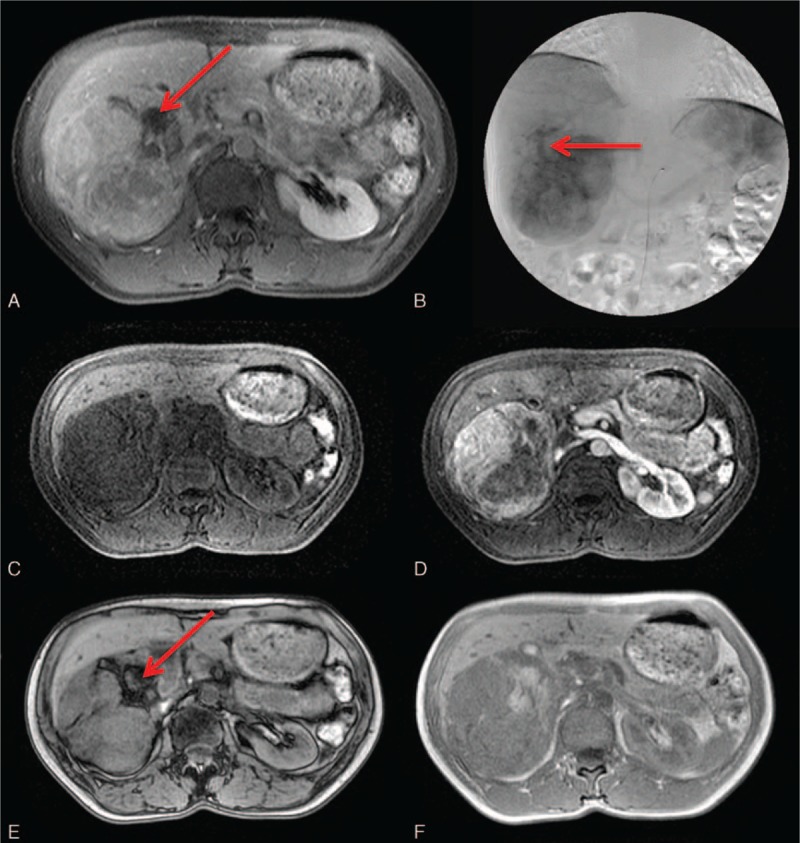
A 40-year-old woman with a hepatic angiomyolipoma (AML). No significant discomfort was noted. A, The presence of macroscopic fat is clearly detected as low signal (arrow) by T1-weighted MRI with fat suppression. B, Angiography shows a draining vein (arrow) from the center of the tumor mass; this is a key characteristic of AML. Pre- (C) and post- (D) contrast T1-weighted images depict the inhomogeneous enhancement due to various degrees of angiomyomatous contents. On dual gradient-echo images, a chemical shift artifact is observed in the cancellation of the signal (arrow) on the out-of-phase (E), compared with the in-phase image (F). MRI = magnetic resonance imaging.
